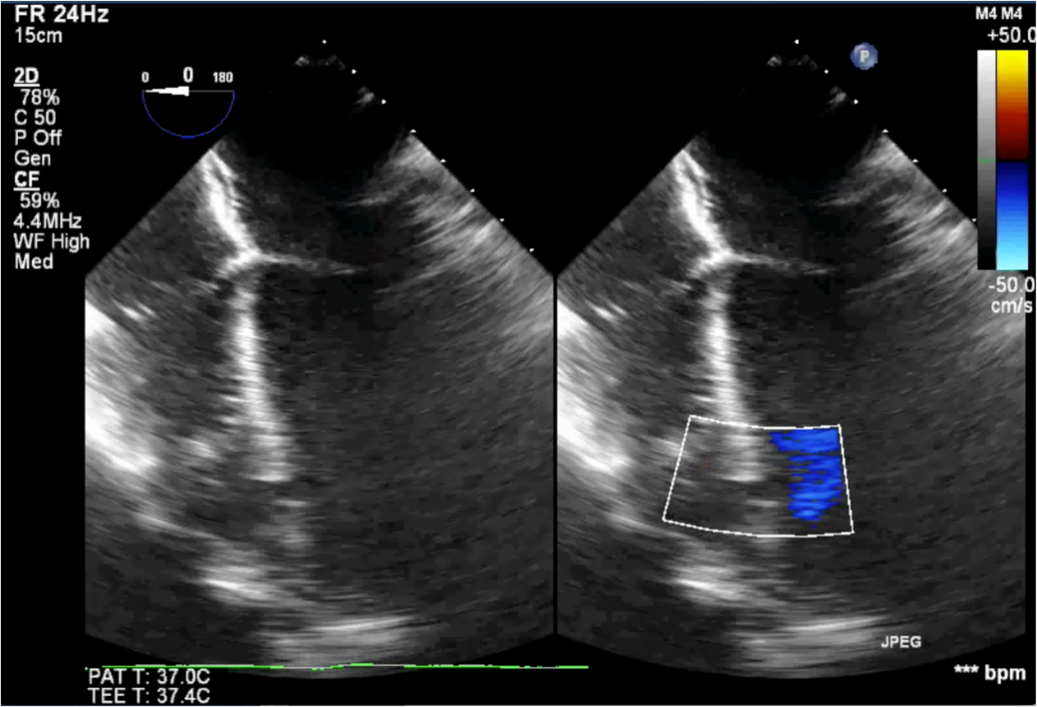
Intra-operative TOE images demonstrating absence of colour doppler flow across region of VSD after direct surgical closure of the defect.
